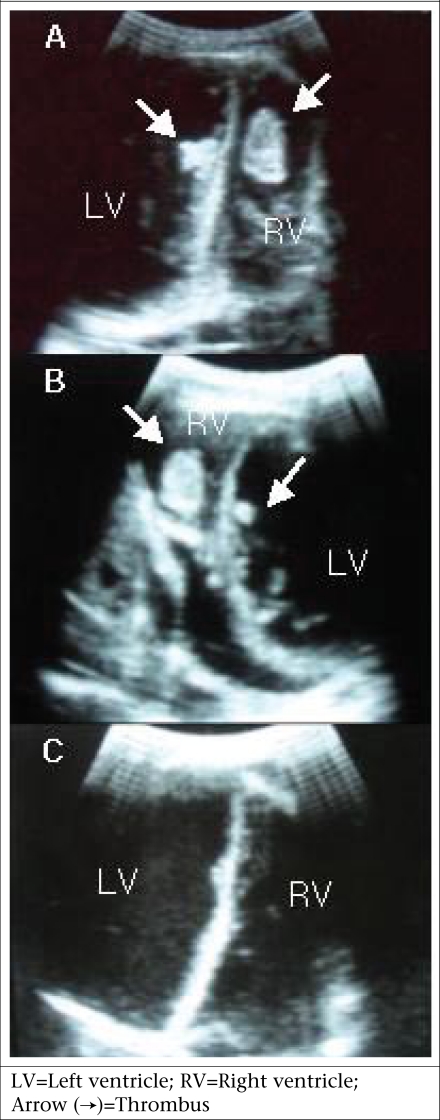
Echocardiogram showing biventricular thrombi (A, B), and the thrombi had disappeared on the 21st day after anticoagulation (C)
